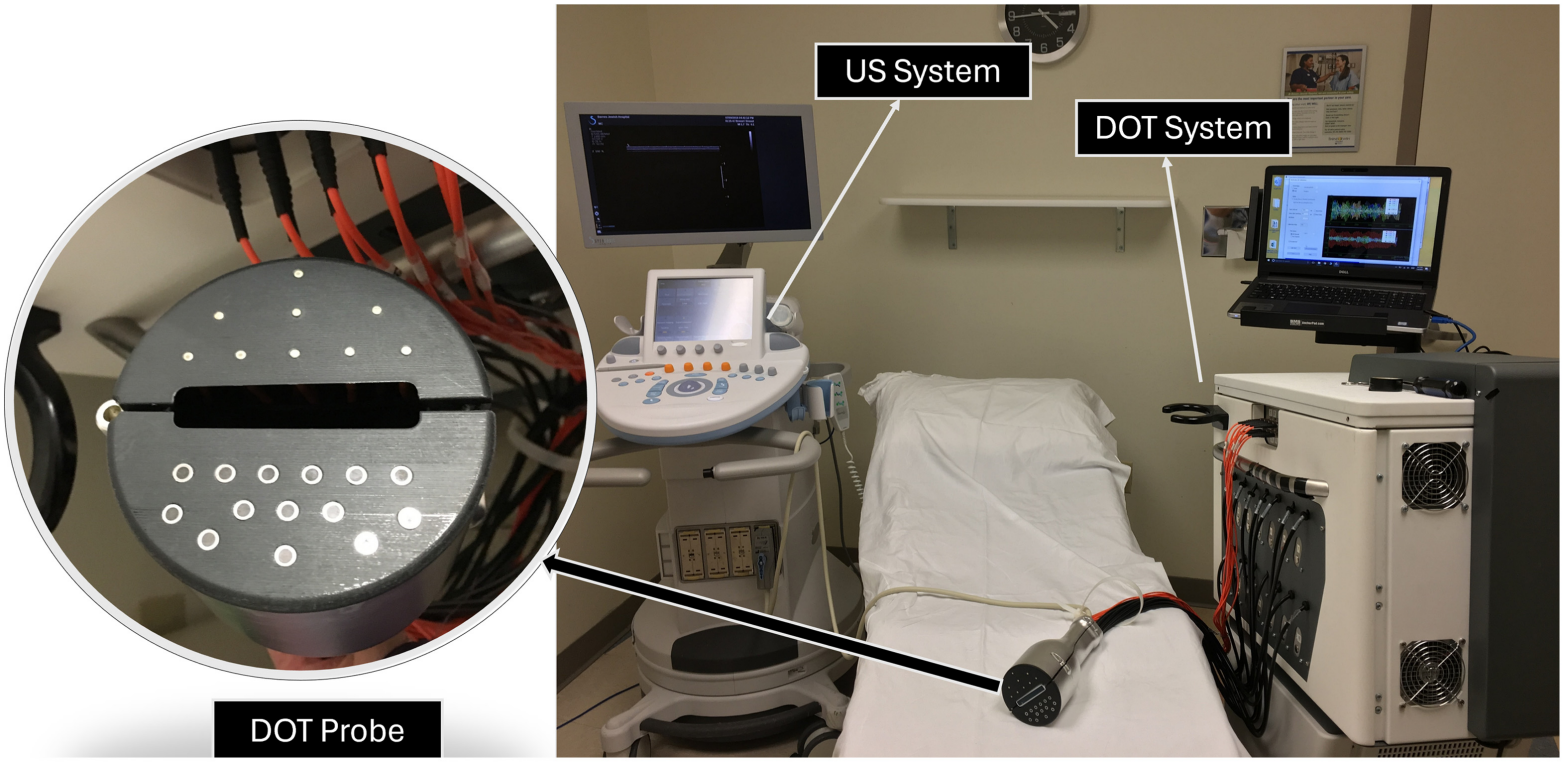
Ultrasound-guided diffused optical tomography system. The handheld probe is shown on the left.
